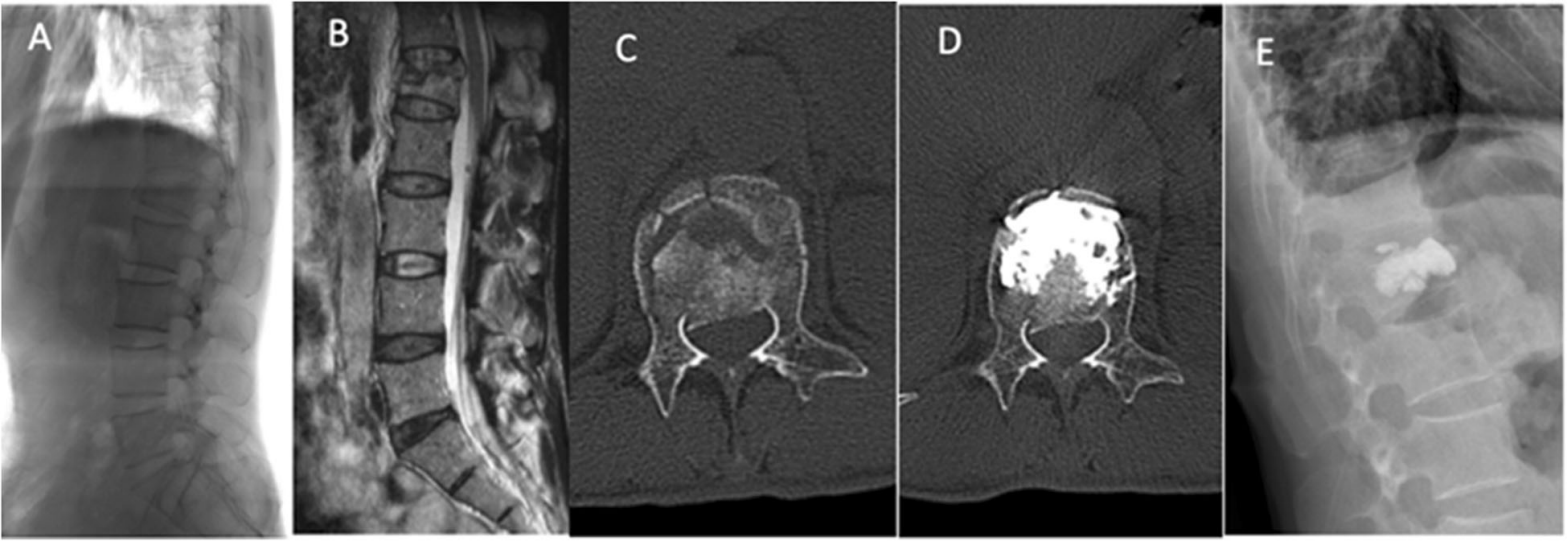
Images of patient #2. a: X-ray radiographs before surgery; b: preoperative weighted MRI image of T2; c: axial CT image of L1; d: postoperative CT image shows that most of the fracture line is filled with cement without leakage; e: postoperative X-ray shows that the fracture line is filled with cement without leakage
